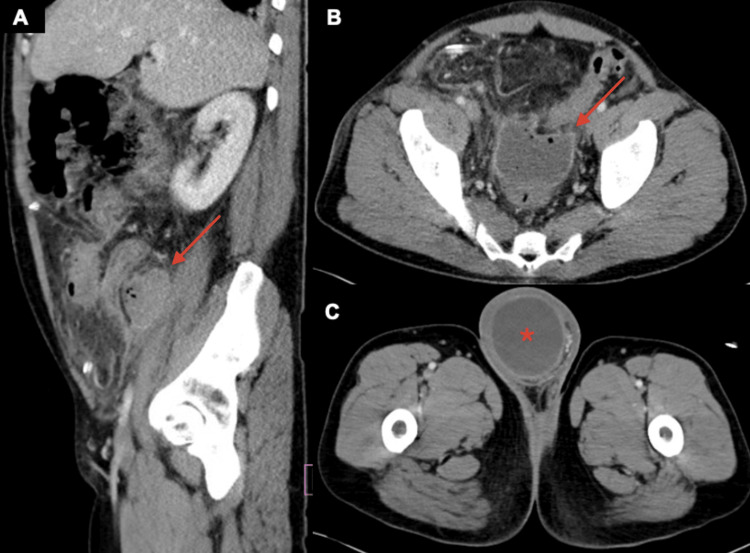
Sagittal (A) and axial (B and C) CT images of the abdomen and pelvis with oral and intravenous contrast demonstrating a 3.5 x 4.9 cm right psoas muscle abscess (A, red arrow), a 4.4 x 6.6 cm deep pelvic abscess (B, red arrow), and fluid extending into the scrotum via a patent inguinal canal, suggestive of abscess versus reactive hydrocele (C, red asterisk) CT: computed tomography
